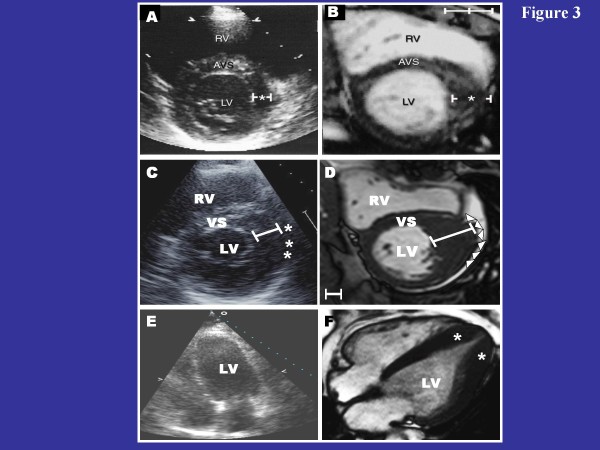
CMR can identify segmental LV hypertrophy that may not be reliably visualized by two-dimensional echocardiography. (A) normal 2-D echocardiogram in a patient with a family history of HCM; (B) this same patient then underwent CMR, which reveals an area of segmental hypertrophy in the anterolateral LV wall (asterisk) consistent with a diagnosis of HCM. Reproduced with permission of American Heart Association; from Rickers C et al.;[35] (C) two-dimensional echocardiographic end-diastolic basal short-axis view demonstrates a maximal LV wall thickness of 18 mm in the anterolateral free wall consistent with the diagnosis of HCM; (D) in the same patient, an end-diastolic short-axis CMR at the same level of LV shows a focal area of massive LV hypertrophy (35 mm) in the same region of the LV wall reported to be 18 mm by echocardiography. The finding of massive hypertrophy by CMR, characterized this patient as high risk, and prompted recommendation for ICD therapy for primary prevention of sudden death. Reproduced with permission, from Maron MS et al.;[42](E) echocardiography was considered non-diagnostic; (F) in the same patient, CMR clearly demonstrates segmental hypertrophy confined to the LV apex, consistent with a diagnosis of apical HCM. Reproduced with permission, from Moon et al.[43] LV = left ventricle; RV = right ventricle; VS = ventricular septum.
